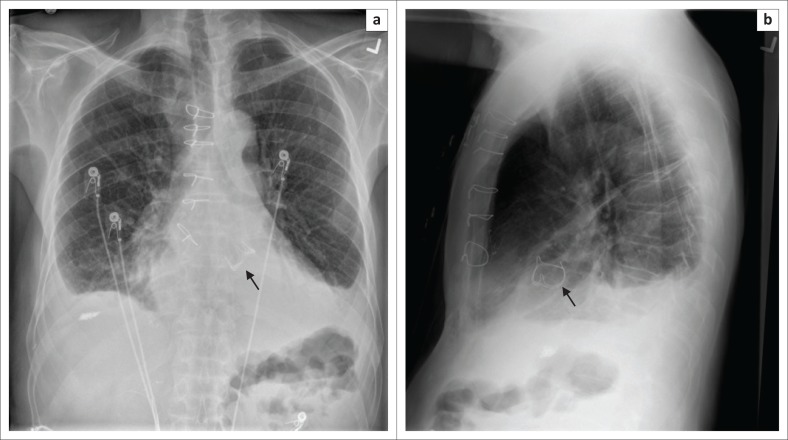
Frontal (a) and lateral (b) chest radiographs showing an aortic valve (perimount magna ease tissue valve) replacement (arrow) in a 76-year-old male patient with severe aortic stenosis.
